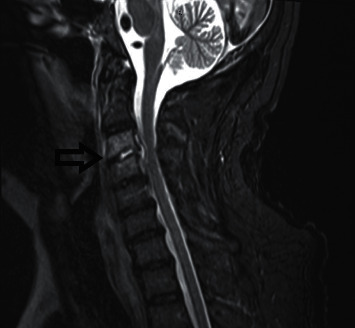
MRI of spine with and without contrast showing C_3_-C_4_ discitis-osteomyelitis (black arrow), with associated epidural abscess (day 9 of hospitalization).
